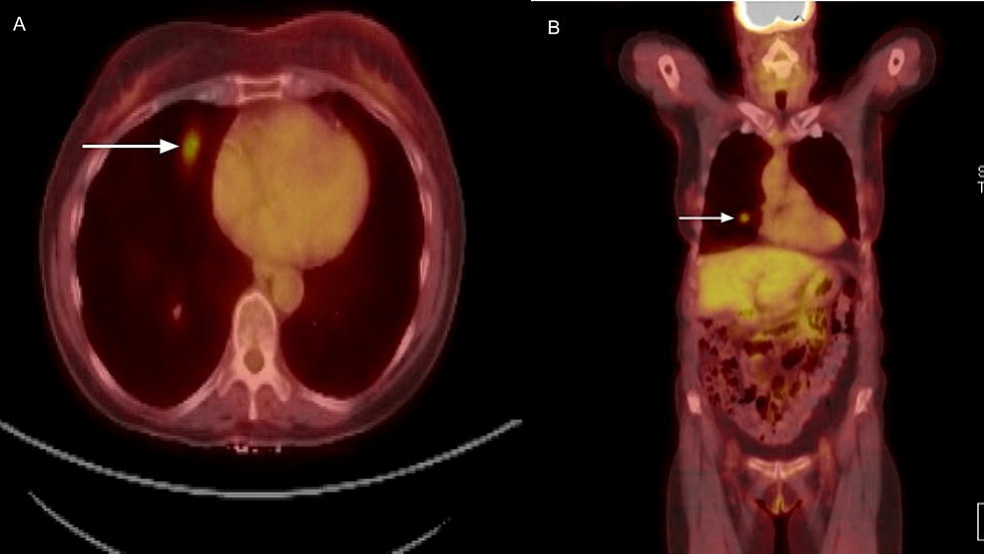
PET-CT showed focal activity with a standardized uptake value (SUV) maximum of 5.5 localizing to the suspicious right middle lobe lung nodule (A: axial view, B: coronal view). PET-CT: Positron emission tomography-computed tomography.
